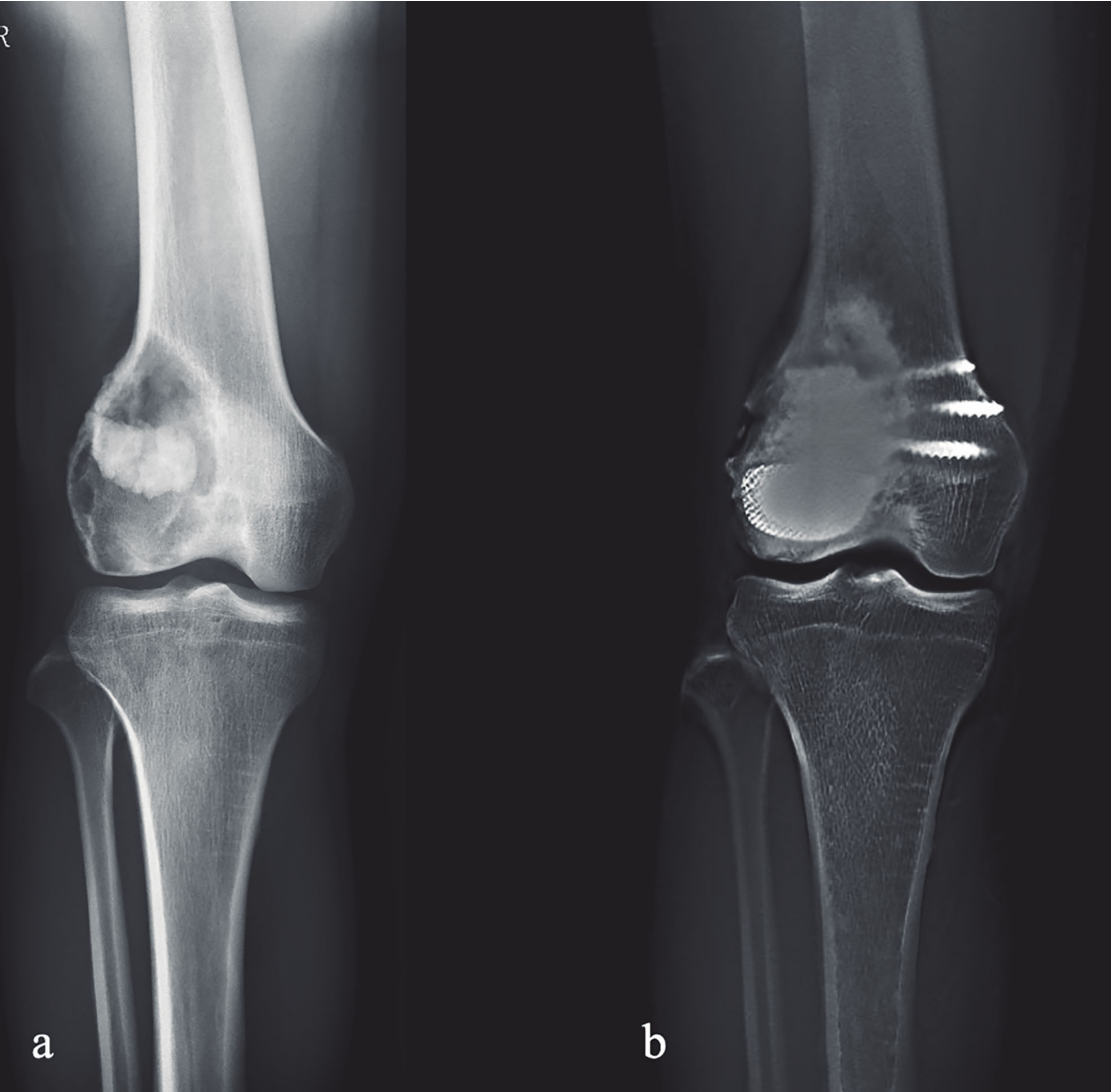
Preoperative and postoperative X-ray evaluations: (A) Preoperative AP view of one patient from the cohort. (B) Extended curettage, subchondral bone grafting and 3D-printed strut-type prosthetic reconstruction were performed; T-SMART taken at 24 months after surgery showed that the subchondral bone thickness was increased and osseointegration was achieved.
